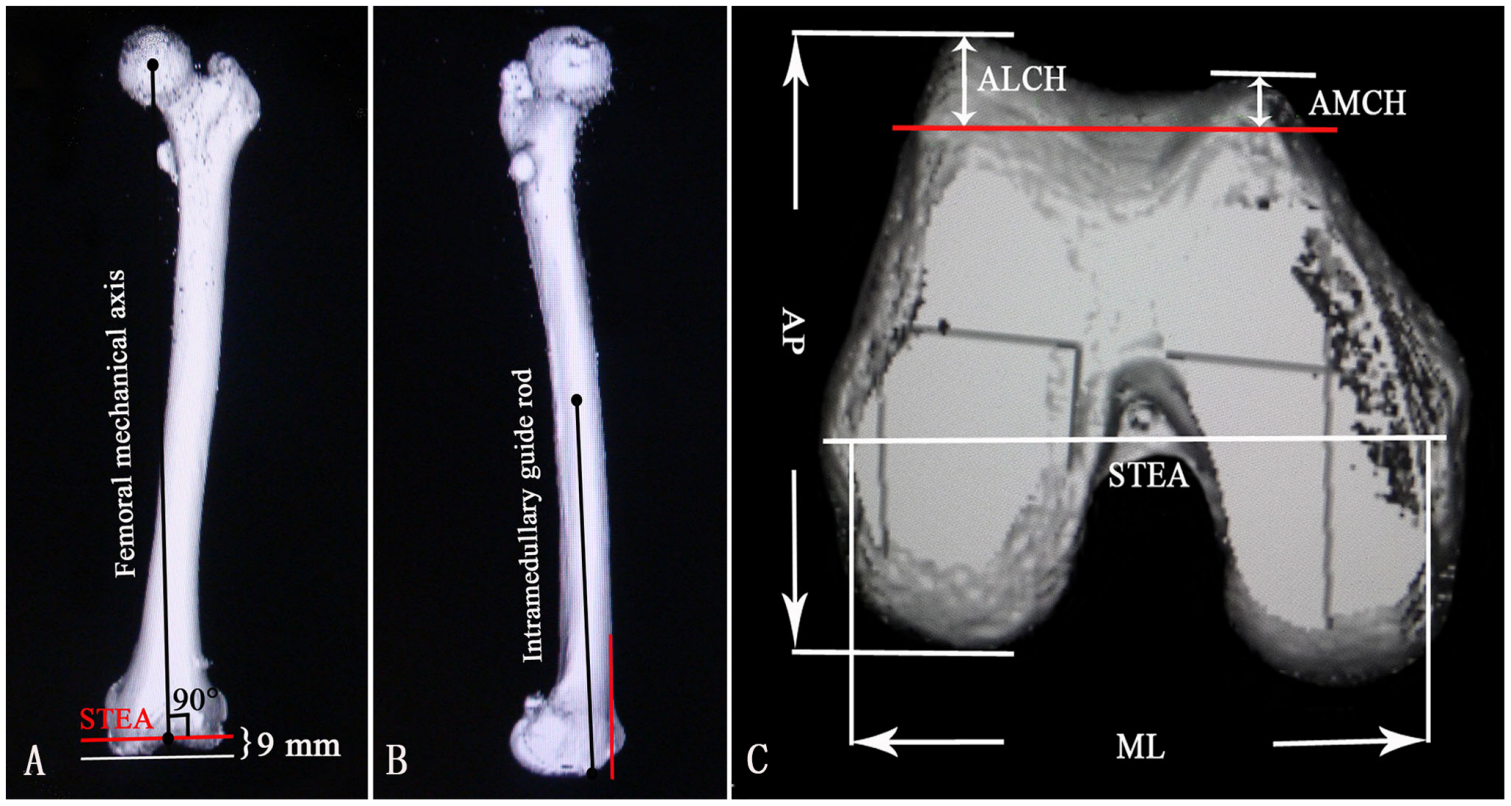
Cuts and measurements of the distal femur on computed tomography (CT) images. (A) A coronal CT image of a femur. The red line indicates the distal femur cut line. (B) A sagittal CT image of a femur. The red line indicates the anterior condyle cut line, which is flush with the anterior femoral cortex. (C) An axial CT image shows the measurement parameters for the distal femur. The red line indicates the anterior condyle cut line.
